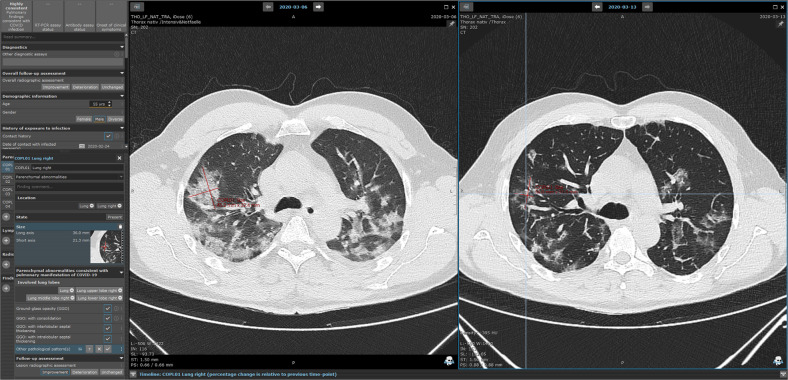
COVID-19 EDC on the mint Lesion^TM^ software platform. In this example, the patient had confirmed COVID-19 and underwent chest CT at two subsequent time points. The images were assessed and evaluated using the Mint EDC by an experienced radiologist. The assessment and evaluation are performed directly on primary imaging data supported by the automatic rule-based evaluation of disease progression. The patient was included in our feasibility clinical trial directly without the necessity for further data collection or redundant documentation.
